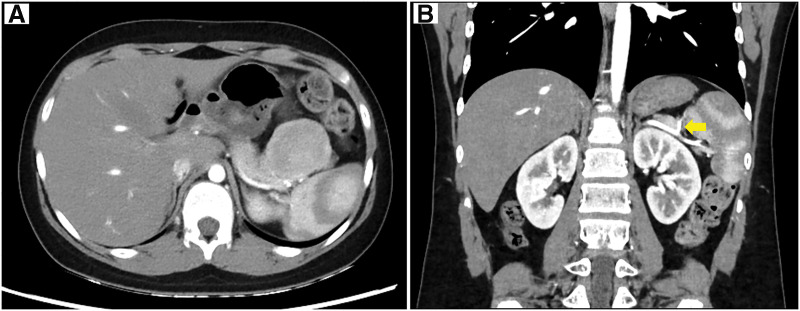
Abdominal CT. (A) Abdominal CT revealed a 6-cm nodular lesion in the anterior part of the pancreatic tail with strong contrast. (B) The tumor-feeding artery (arrow) branched off from the splenic artery.
